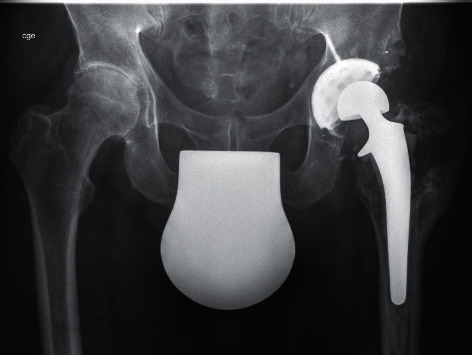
X-ray prior to the third revision surgery (2020).
